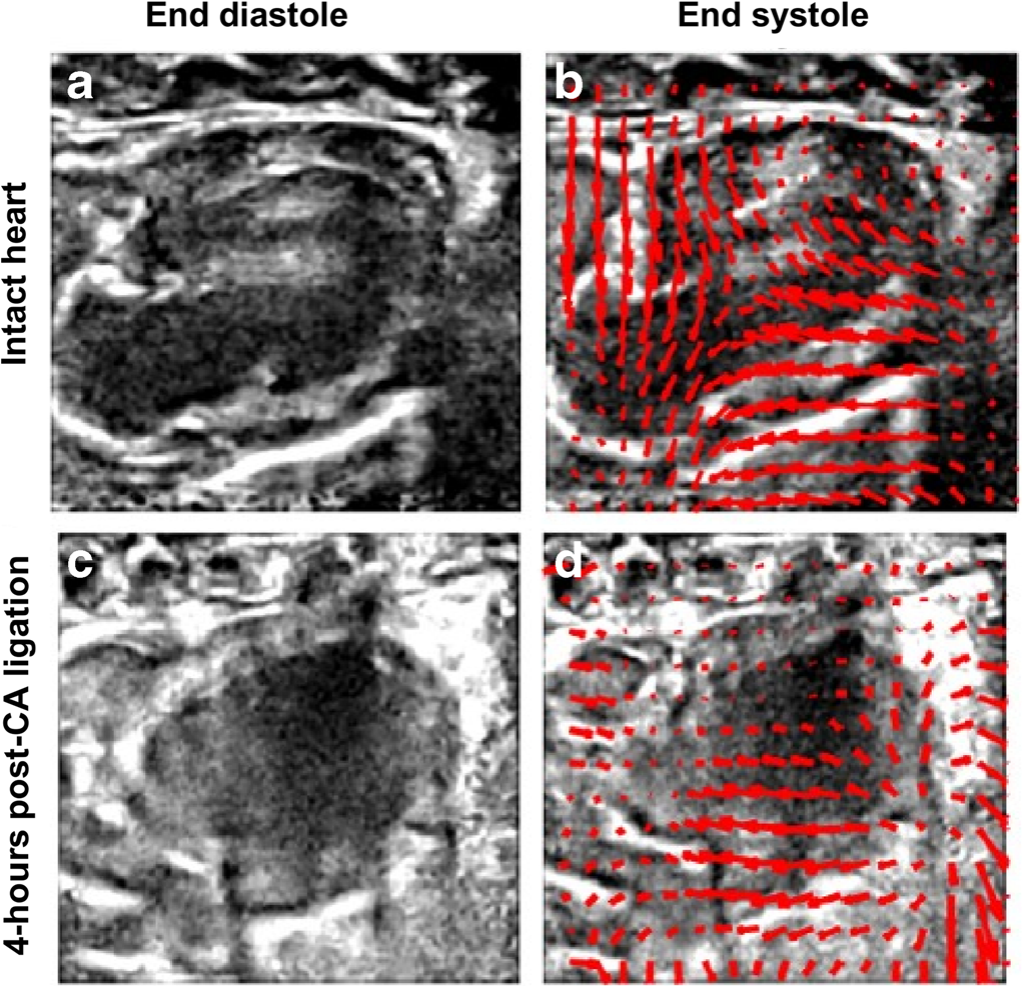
Fusion of B-mode ultrasound images and motion vector field (as red arrows) of the cardiac deformation from end-diastole (a, c) to end-systole phases (b, d). Panels a and b represent an intact heart and panels c and d 4-h after left-descending coronary artery ligation.
